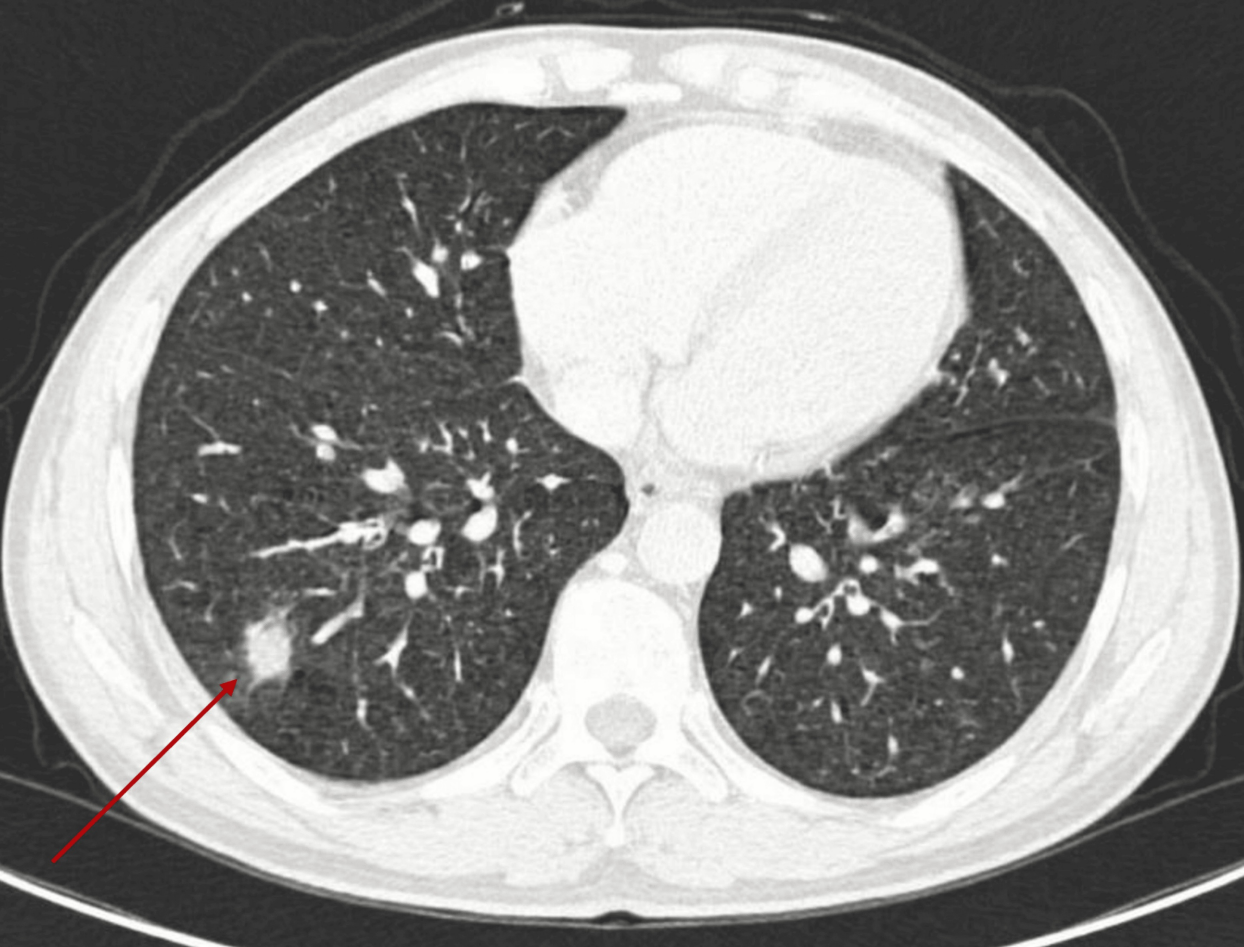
Chest CT demonstrating pulmonary nodule. Axial chest CT image obtained during initial staging showing pulmonary nodule in the lower lobes (red arrow), suspicious for metastatic involvement in the context of malignant peripheral nerve sheath tumor.
